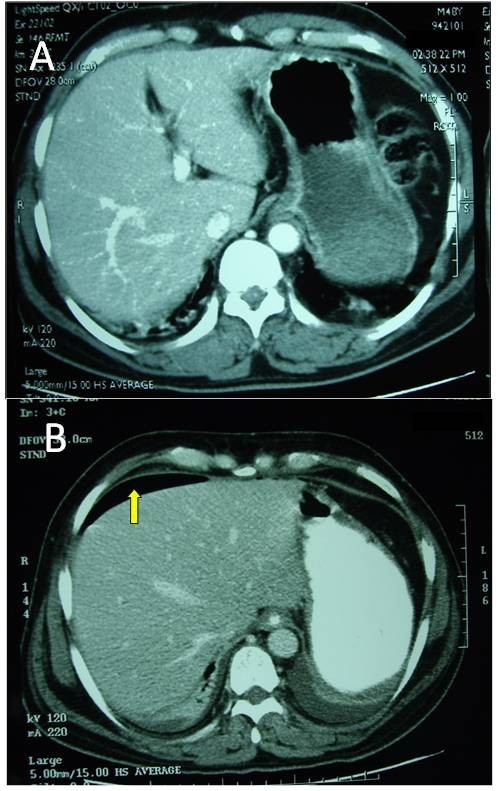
Abdominal CT scan with intravenous contrast on day 1 (A) which was normal and on day 3 (B) which showed free intraperitoneal air (arrow) and left pleural effusion.
